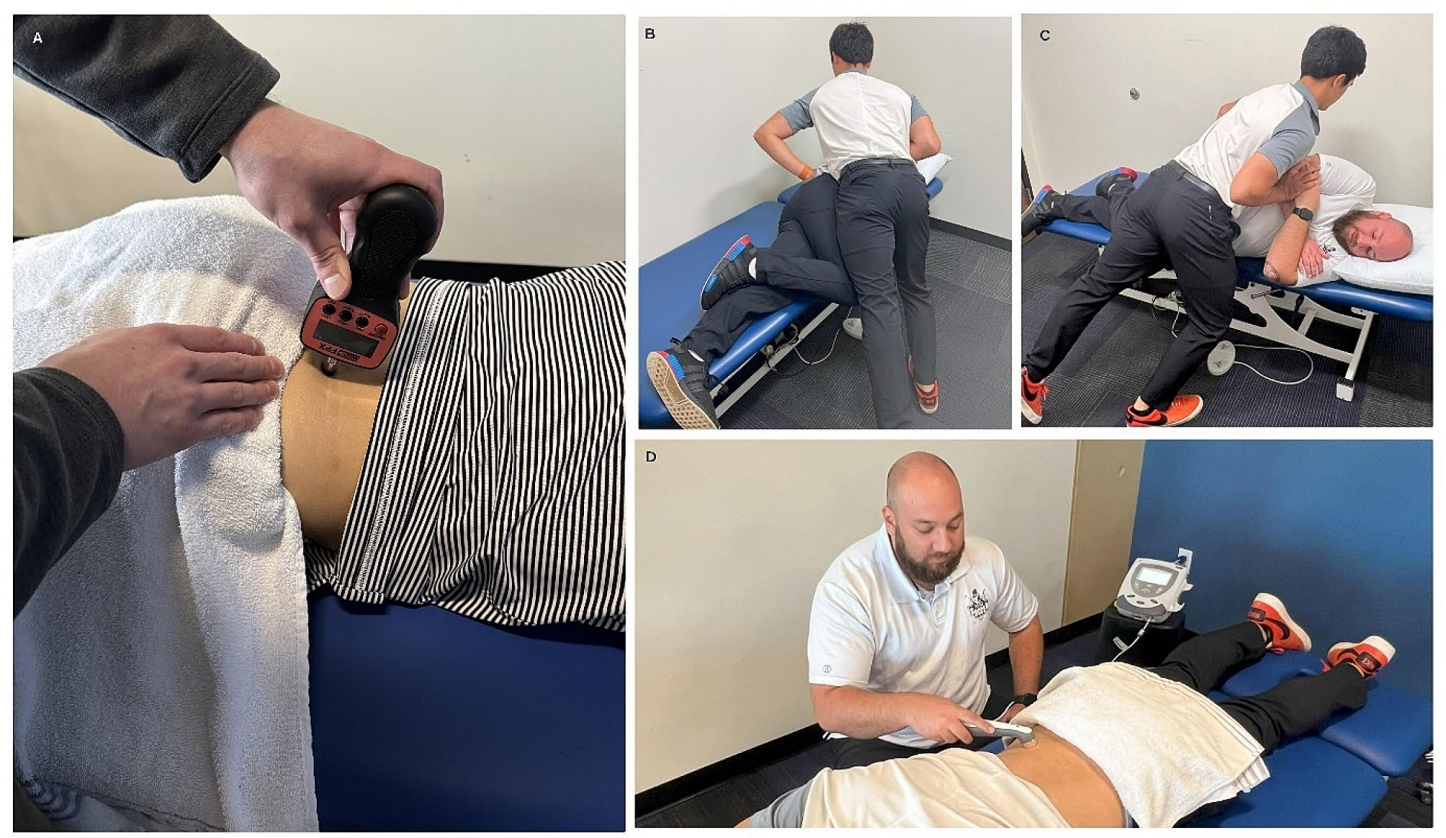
(A) Assessment of pain pressure threshhold at the posterior superior iliac spine using the algometer, Interventions performed with patient positioning for the (B) sidelying lumbar manipulation from the side, (C) sidelying lumbar manipulation from a posterior view, and (D) deactivated ultrasound to the posterior superior iliac spine.
